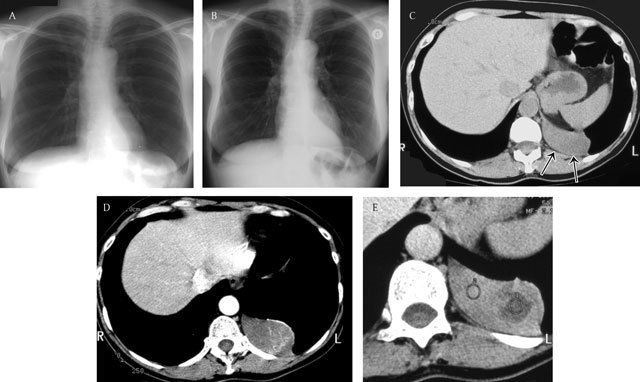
Localised fibrous tumor of the pleura – Two consecutive chest radiographs (a, b) with an interval of 3 years show a sharply delineated slow growing retrocardiac mass. Unenhanced axial CT image (c) shows a well-defined homogeneous soft-tissue-density mass. Note the intact subpleural fat (black arrows). Enhanced CT images (d, e) show heterogeneous enhancement corresponding to areas of necrosis. (Courtesy of G. Ferreti, Grenoble, France).
